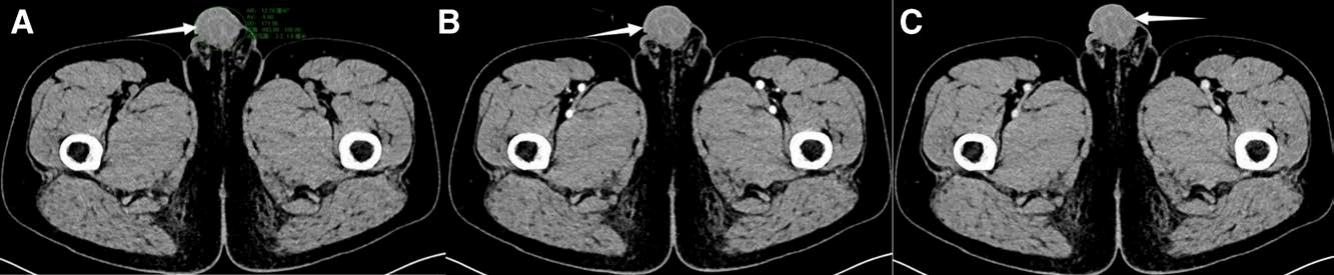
CT showed an obvious mass in the scrotum (A), and the enhanced scans: (B) arterial phase, (C) venous phase showed no enhancement.
